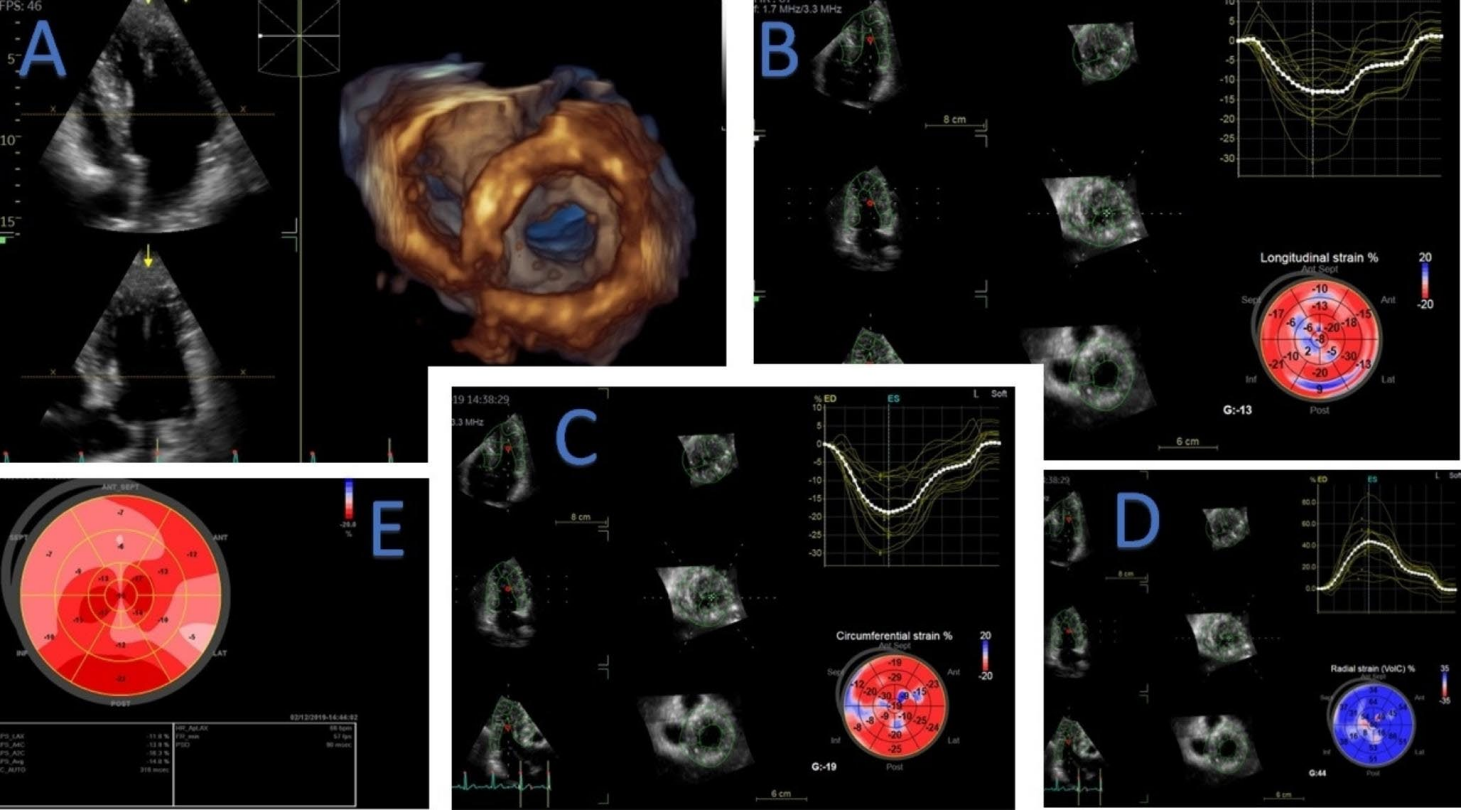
Echocardiographic evaluation of the same patient: 3D echocardiography representation (A), 3D GLS (B), 3D GCS (C), 2D GRS (D) and 2D GLS (E)
